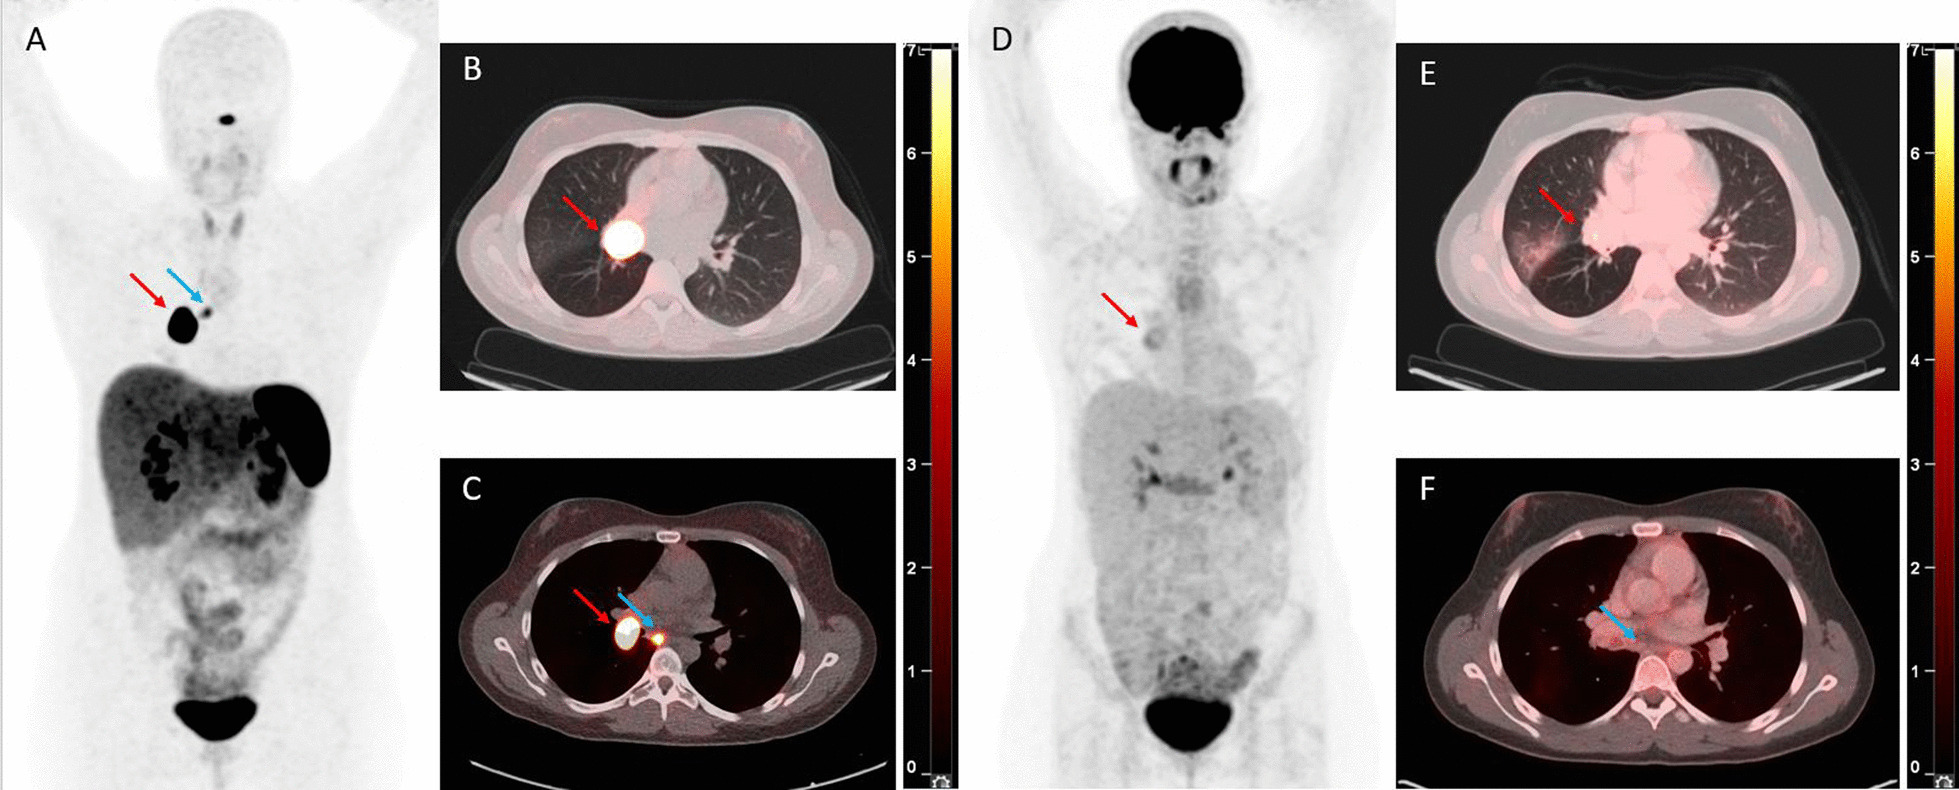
21-year-old patient with a typical bronchial carcinoid (TC) who underwent a [^68^Ga]Ga-DOTATOC PET/CT scan showing two foci of intense tracer uptake on the MIP image (A) corresponding to the primary hilar tumour (red arrow, B) and to an infracarinal nodal metastasis (blue arrow, C), as well as an [^18^F]FDG PET/CT scan showing only a limited tracer uptake in the tumour (red arrow, visible on the MIP image (A) and an axial fusion image (E)) and no increased tracer uptake in the infracarinal nodal metastasis (blue arrow, F). The SUV_max_ values on [^68^Ga]Ga-DOTATOC PET/CT were 110 and 9.2 in the tumour and in the infracarinal lymph node, respectively, whereas those on [^18^F]FDG PET/CT were 4.7 and 2.3 in the tumour and the infracarinal lymph node, respectively
